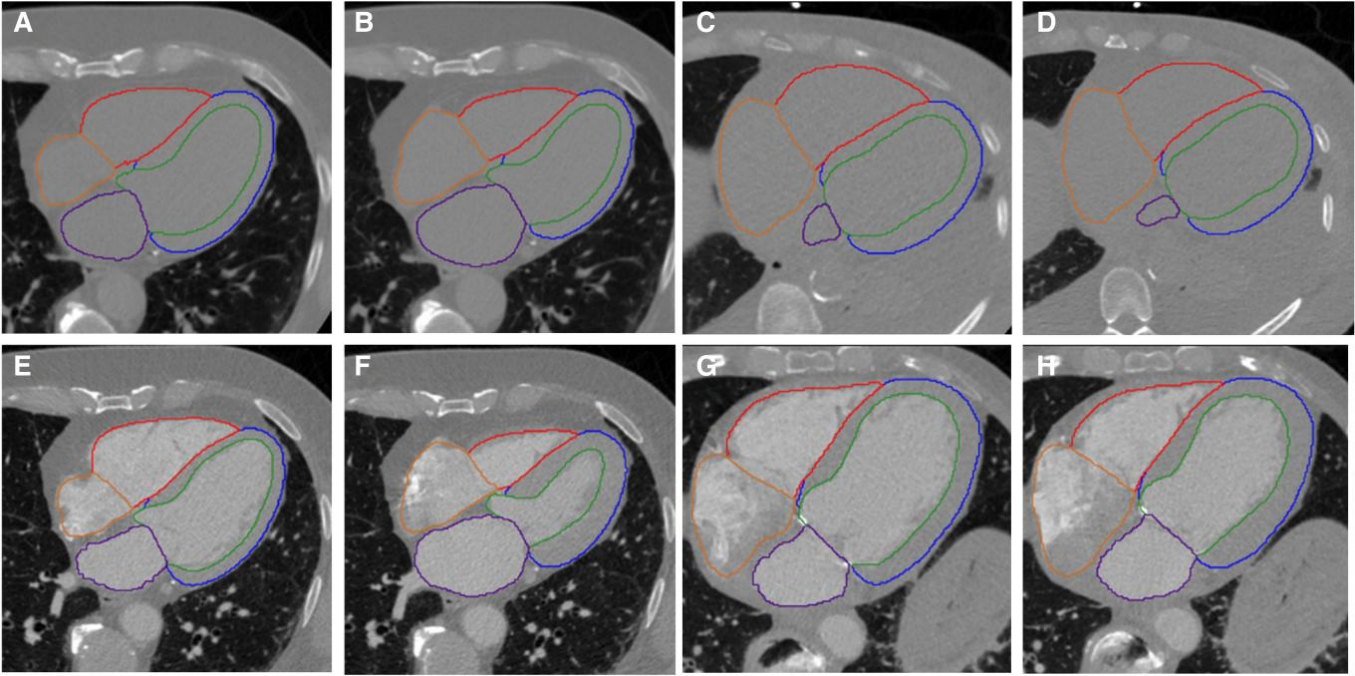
AI-based segmentation of the left and RVs and derived EF. (*A–D*) Chamber segmentation in NCCT, (*E–H*) chamber segmentation in CCT. Panels (*A, B*) and (*E, F*) show a patient with an EF above 60% in CCT, while panels (*C, D*) and (*G, H*) show a patient with an EF below 50% in CCT. NCCT, non-contrast cardiac CT; CCT, contrast cardiac CT.
